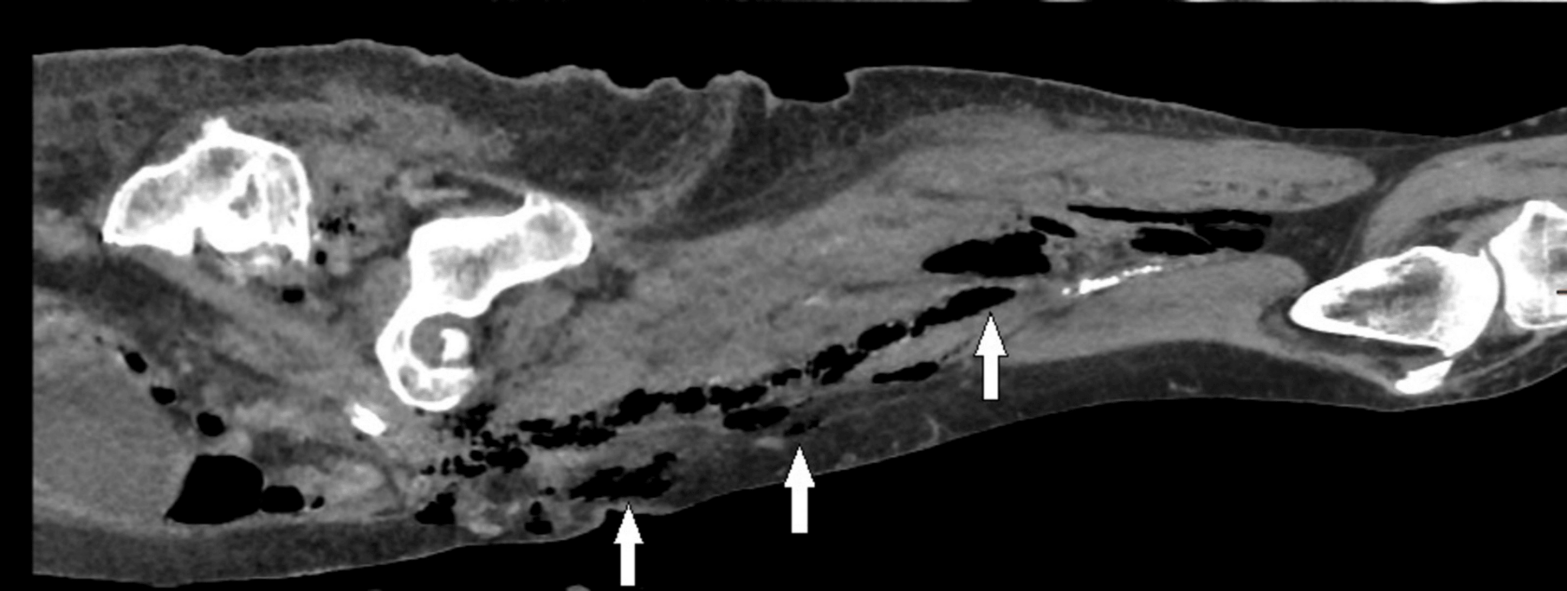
Sagittal plane CT scan of the right thigh showing tracking of surgical emphysema.
